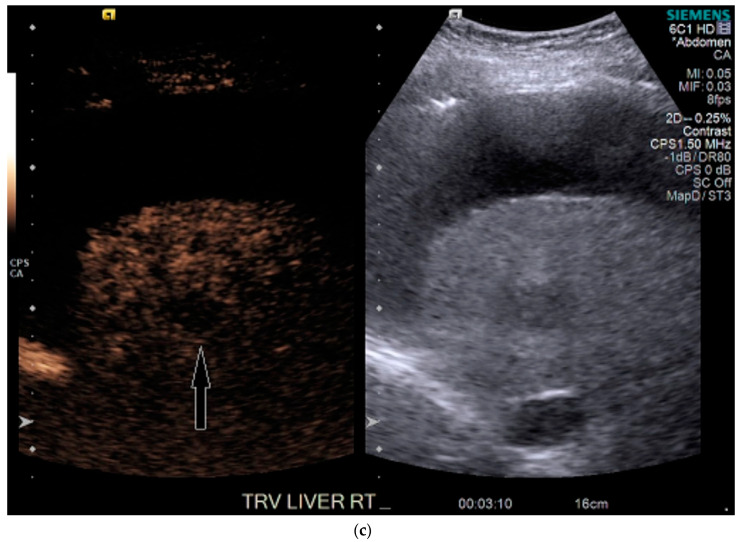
Patient 3 ultrasound images, ascites is present with lesion denoted by white arrow: (a) grayscale images showing the hypoechoic lesion. Post contrast administration ultrasound in arterial phase shows subtle enhancement compared with liver parenchyma at the same level (b) and delayed phase mild washout (c) of the lesion, LI-RADS 5.
